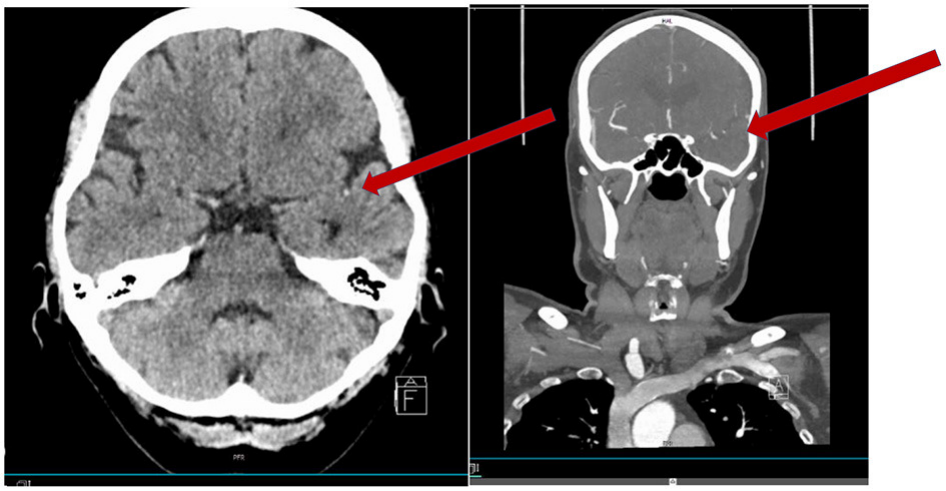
Fifty-nine-year-old woman with an acute onset of aphasia (NIHSS 1 disabling). CT scan showing a hyperdense vessel sign in M2 (arrow). CTA showing the occlusion in M2 (short in length).
